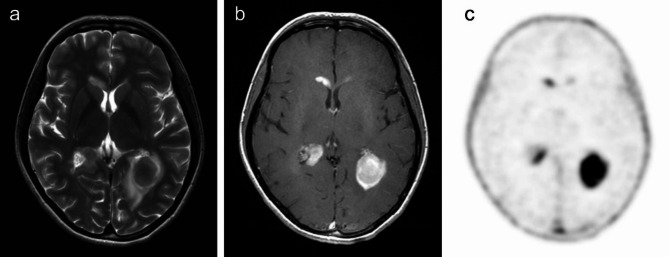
[^18^F]FLT-PET/MRI in a patient with an atypical variant of a tumefactive multiple sclerosis verified by a biopsy. Multiple contrast-enhancing lesions (b) with relatively low signal intensity on a T2-weighted image in the axial plane (a) are present in the periventricular white matter of both hemispheres, the largest one on the left side near the trigone of the lateral ventricle with a hyperintense rim of perifocal oedema. All lesions demonstrate very high uptake of [^18^F]FLT (c). PET examination in this case failed to rule out tumorous aetiology (e.g. lymphoma), which was considered due to a very atypical MRI finding
